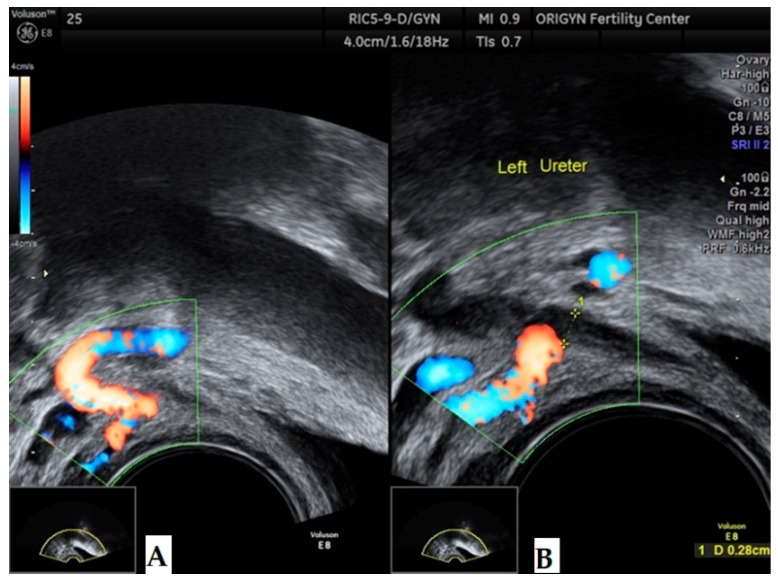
Transvaginal ultrasound of a normal left ureter (A) and of the crossing of the uterine artery when viewed in Doppler mode (B).
